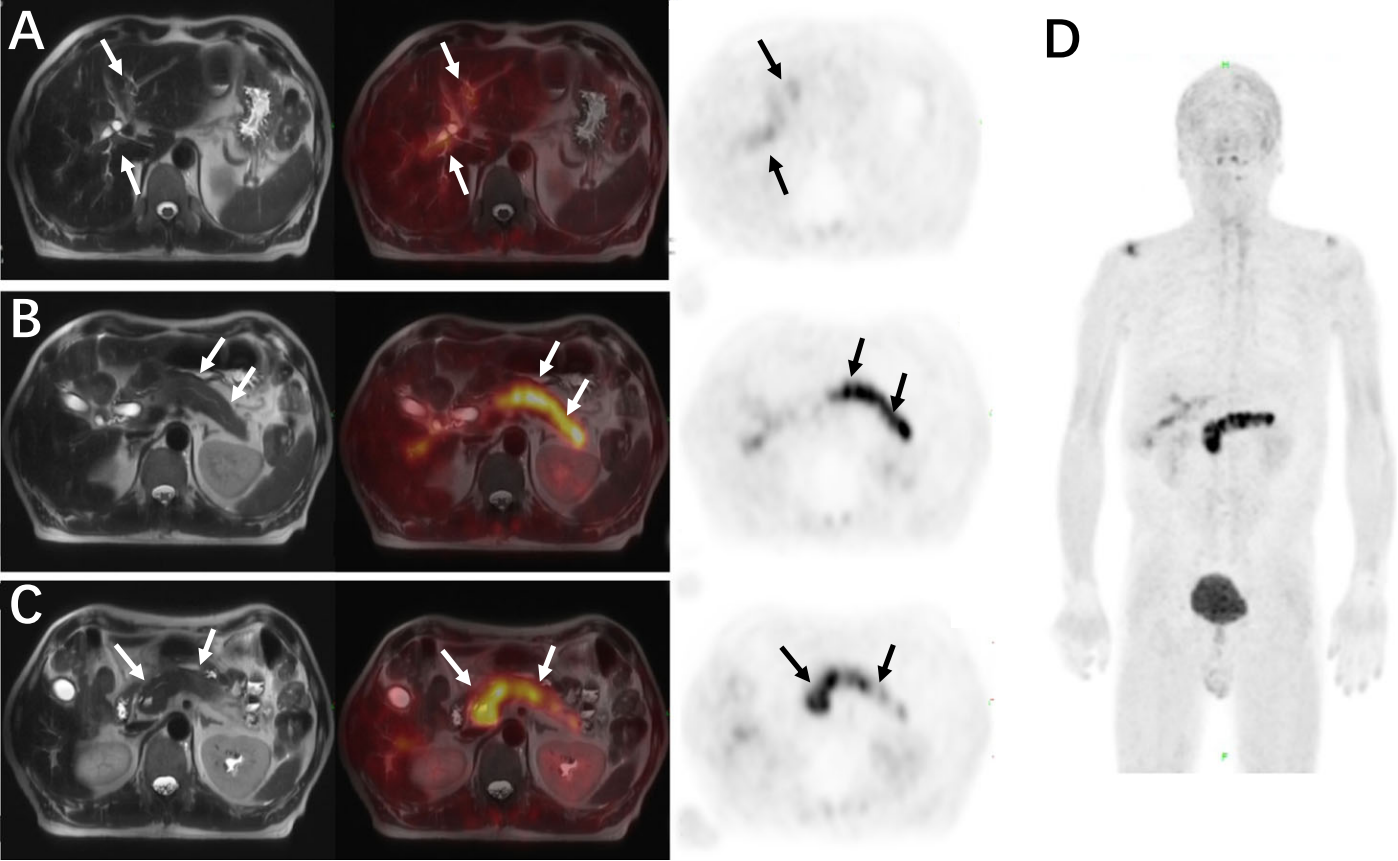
^68^Ga-FAPI PET/MR images of IgG4-RD, the intensely increased ^68^Ga-FAPI uptake in the entire pancreas, and dilated bile duct made unequivocally diagnosis of IgG4-RD
